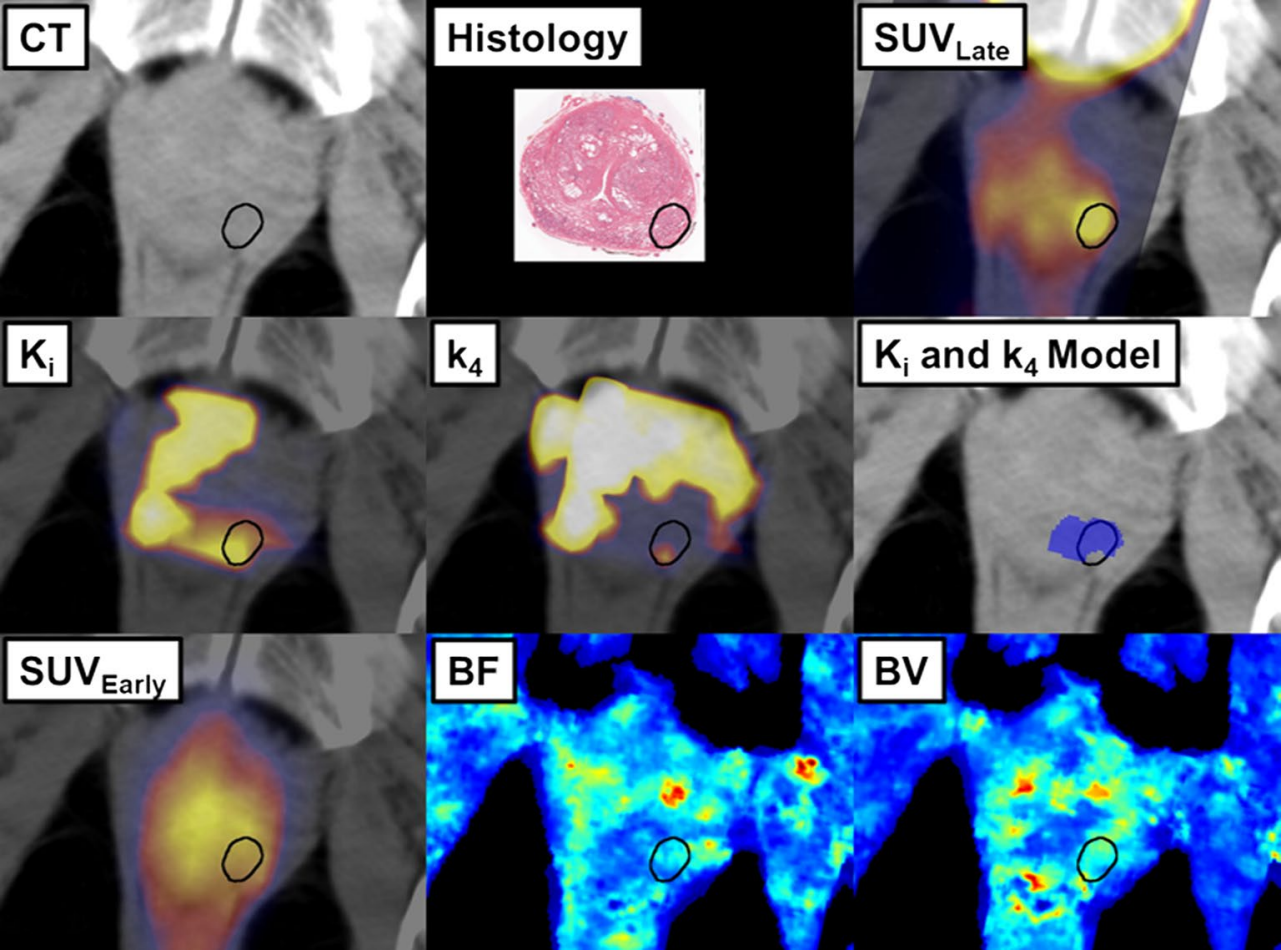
Example of the co-registered in vivo images and parametric maps. The location of DIL is outlined in black. *CT* Computed tomography, *PET* positron emission tomography, *SUV*_*Late*_ 2-h post-injection standardized uptake value, *K*_*i*_ net uptake rate constant, *k*_*4*_ dissociation rate constant, *SUV*_*Early*_ 10-min post-injection standardized uptake value, *BF* blood flow, *BV* blood volume, *DIL* dominant intraprostatic lesion
